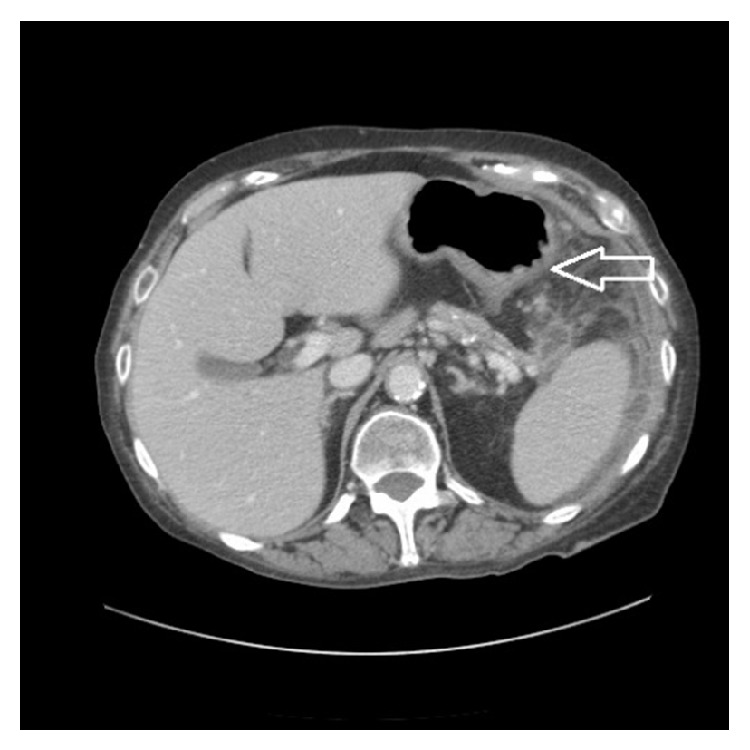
CT abdomen/pelvis done at time of admission to outside hospital showing colonic thickening at the splenic flexure.
